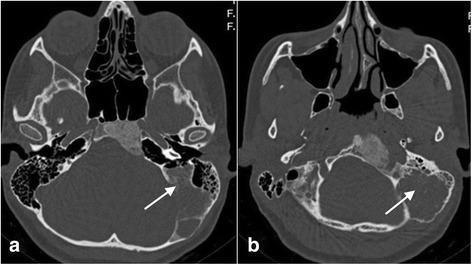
a Axial CT scan at the level of the cochlea demonstrates extensive involvement of the left temporal bone by fibrous dysplasia. b Axial CT scan at the level of the stylomastoid foramen demonstrates extensive involvement of the left temporal bone by fibrous dysplasia
